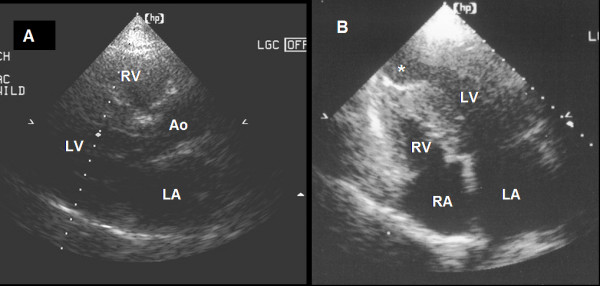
Transthoracic echocardiogram. A: Parasternal long axis view showing asymmetric septal hypertrophic cardiomyopathy in end diastole. B: A four-chamber view showing a combination of basal and mid-ventricular hypertrophic cardiomyopathy and an apical aneurysm (*). (LA = left atrium, RA = right atrium, RV = right ventricle, LV = left ventricle, Ao = aorta).
